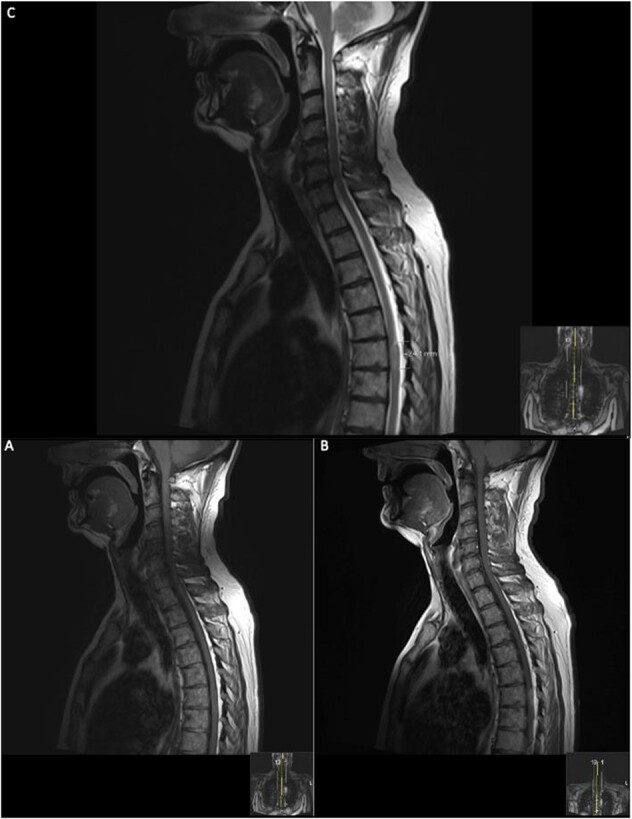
Repeat contrasted MRI whole spine; demonstrates findings consistent with spinal cord meningioma at the level of T6; this can be clearly visualized by comparing the T1 sagittal pre-contrast (A) with a T1 sagittal post-contrast (B), where a clearly demarcated lesion can be seen at the level of T6; this is supported further by the sagittal T2 image (C) shown where an ~24 mm lesion can be seen at T6.
